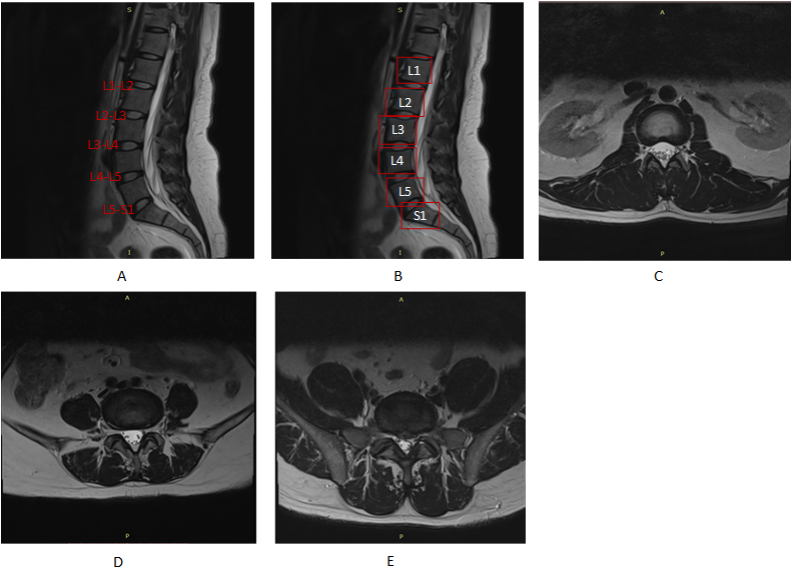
Examples of lumbar MR images. (A) A sagittal lumbar MR image in which 5 IVDs are labeled. (B) A sagittal lumbar MR image in which 6 vertebral bodies are enclosed in boxes. (C) An axial lumbar MR image of a normal disk. (D) An axial lumbar MR image of disk bulge. (E) An axial lumbar MR image of disk herniation. L: lumbar vertebra; MR: magnetic resonance; S: sacral.
